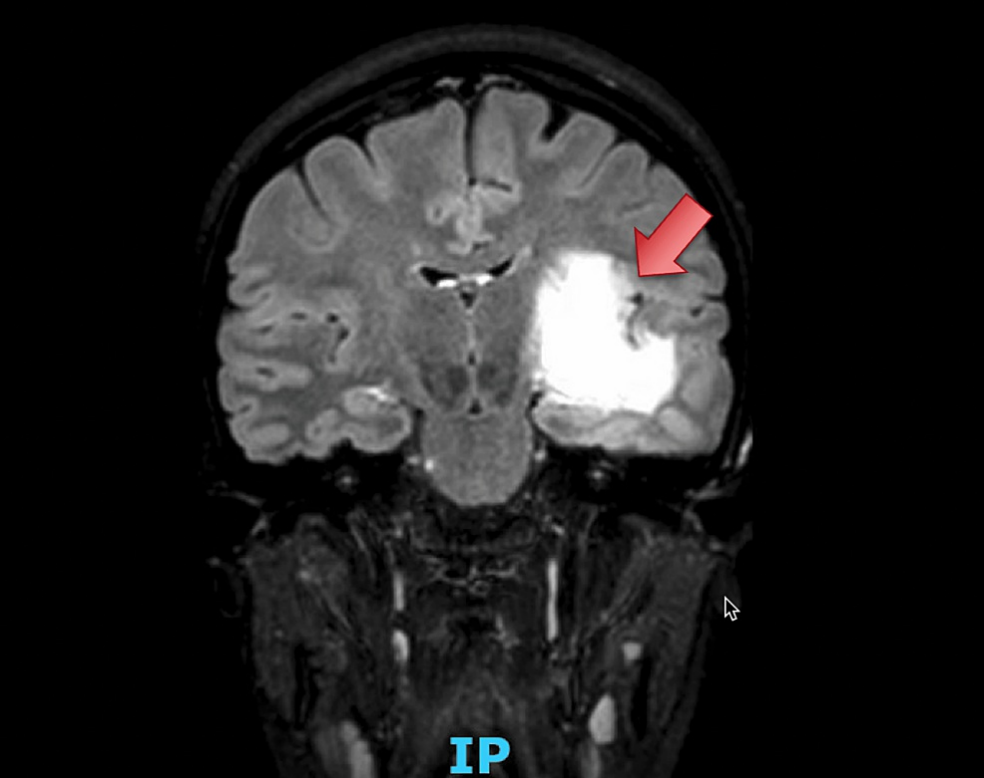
T2 FLAIR frontal sections of brain MRI showing an expansile lesion with surrounding vasogenic edema involving the left temporal stem, posterior subinsular region, posterior basal ganglia, and posterior limb of the internal capsule. FLAIR, fluid-attenuated inversion recovery; MRI, magnetic resonance imaging
